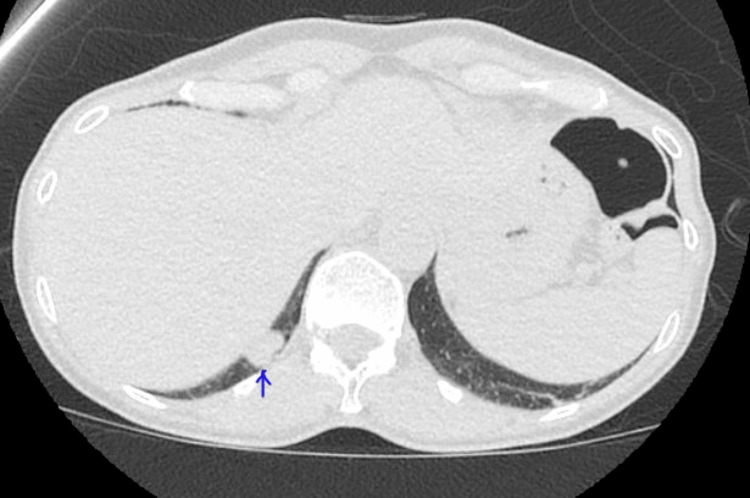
Repeat CT chest a year later showing interval enlargement of the RLL nodule CT: computed tomography; RLL: right lower lobe
